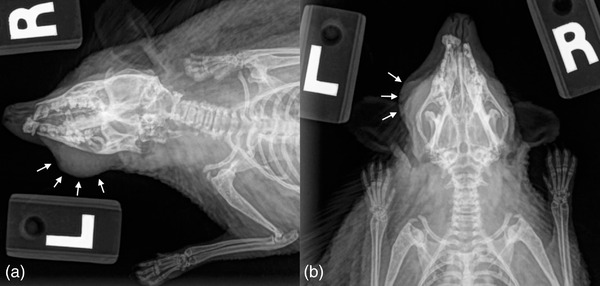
Initial right lateral (a) and dorsoventral (b) radiographs of the hedgehog. There is soft tissue swelling on the lateral aspect of the left maxilla and a displaced rostral maxillary fracture. Additionally, there is a radiolucent region within the caudal left hemimandible adjacent to a soft tissue mass with underlying focal cortical disruption
